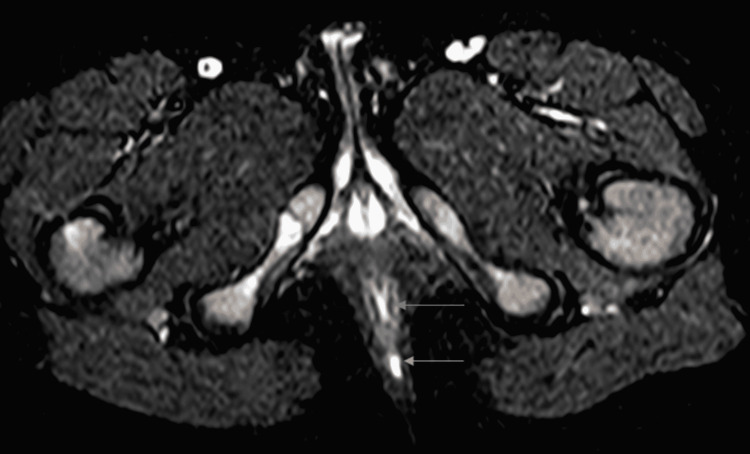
Axial MRI view of the pelvis, showing the true anal canal (blue arrow) and the duplicated anal canal (orange arrow).
